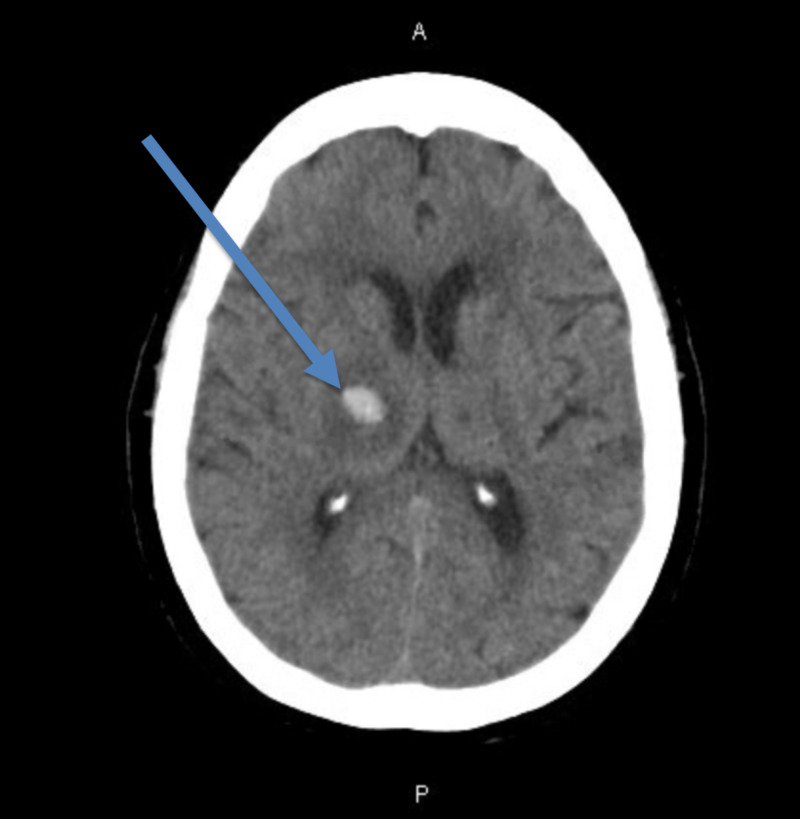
Axial image of CT of brain without intravenous contrast showing hyperdense right sided intra-cranial hemorrhage (blue arrow) that measures 13 mm x 14 mm with surrounding ring of hypodense cytotoxic edema. There is mild mass effect but no associated midline shift.
